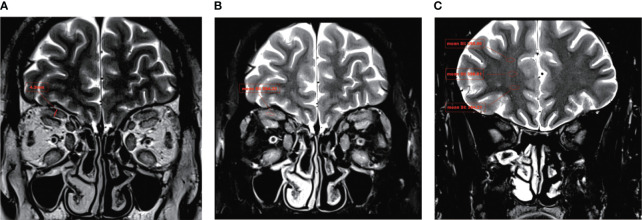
A typical Dixon-T2WI MRI in TAO. In a patient with moderate-to-severe TAO, the T2WI sequence revealed a significant thickening of the LPS and a notable increase in signal intensity. (A) The maximum thickness of the LPS muscle (LPS_T) was measured on coronal in-phase Dixon-T2WI images. (B) The maximum signal intensity of the LPS muscle (LPS_SI) was measured on coronal water-phase Dixon-T2WI images. (C) The signal intensity in ipsilateral brain white matter was measured on coronal water-phase Dixon-T2WI images.
